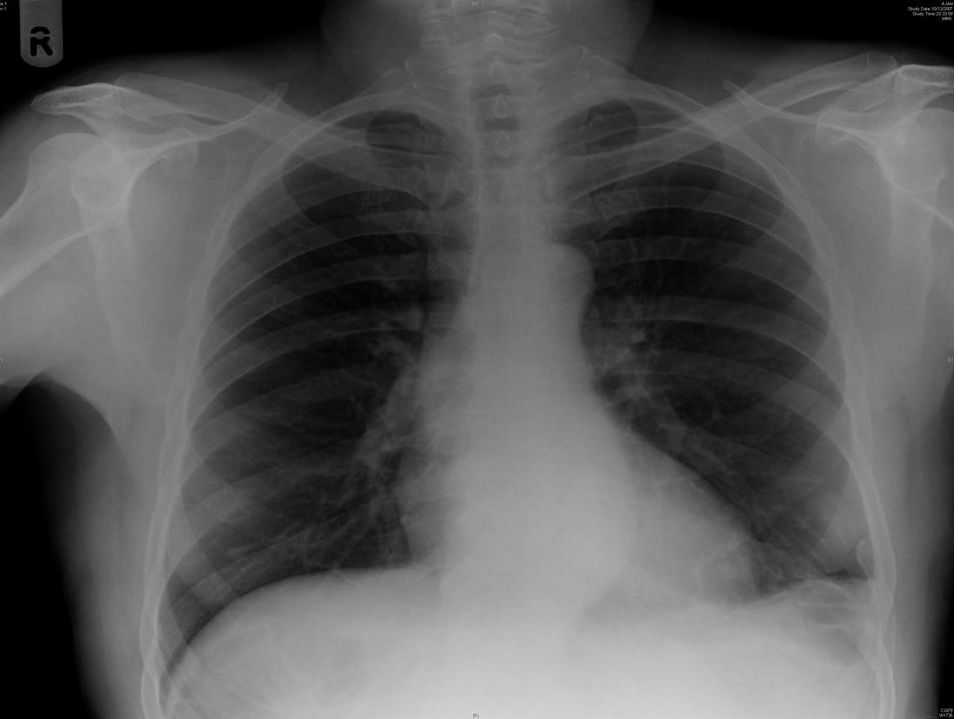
Previous Chest radiograph with a discontinuous left lower posterior 9th rib. Note the normal left hemidiaphragm.
